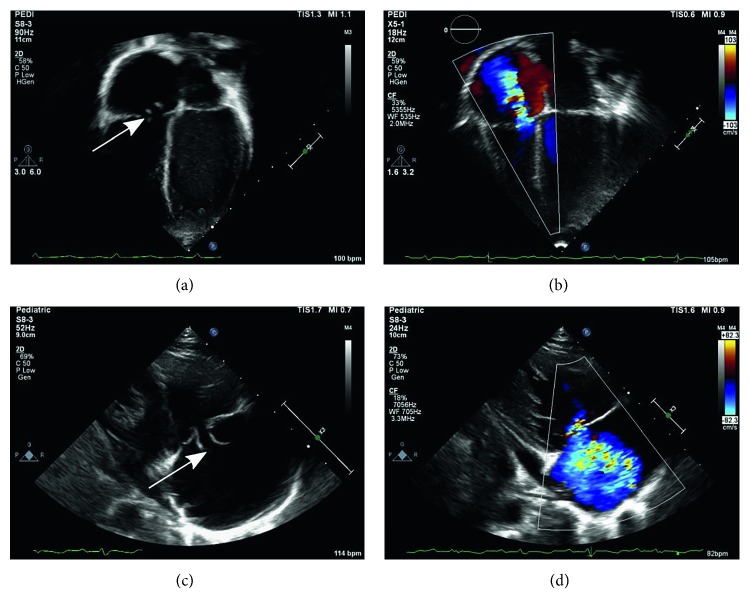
Echocardiogram showing echocardiographic clips: (a) two-dimensional apical 4-chamber view showing tricuspid valve leaflet perforation (arrow) with possible chordal disruption, (b) color Doppler image of apical 4-chamber view showing tricuspid regurgitation (blue jet), (c) two-dimensional parasternal long-axis view showing tricuspid valve leaflet perforation (arrow), and (d) color Doppler image of parasternal long-axis view showing tricuspid regurgitation (blue jet).
